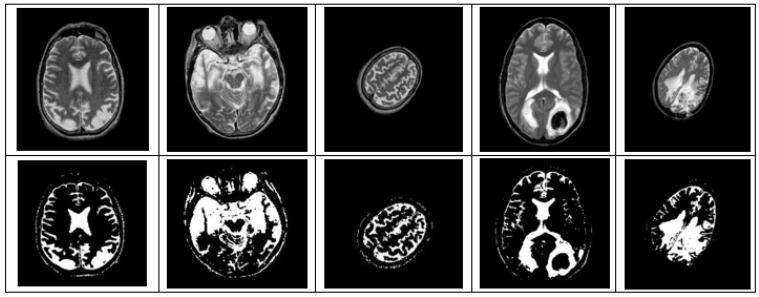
Scaling Invariant (0.25–1.5) Brain MRI Image Detected by CNN.
